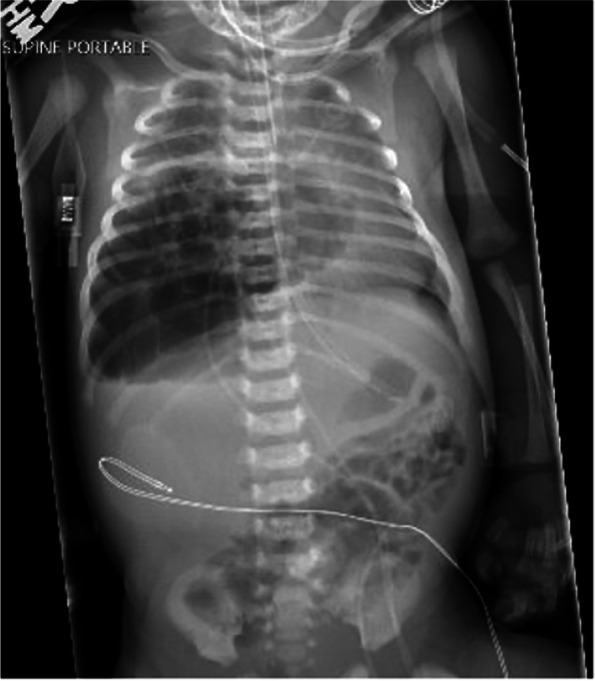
Initial chest radiograph demonstrates a possible congenital diaphragmatic hernia versus multicystic mass causing a left shift
